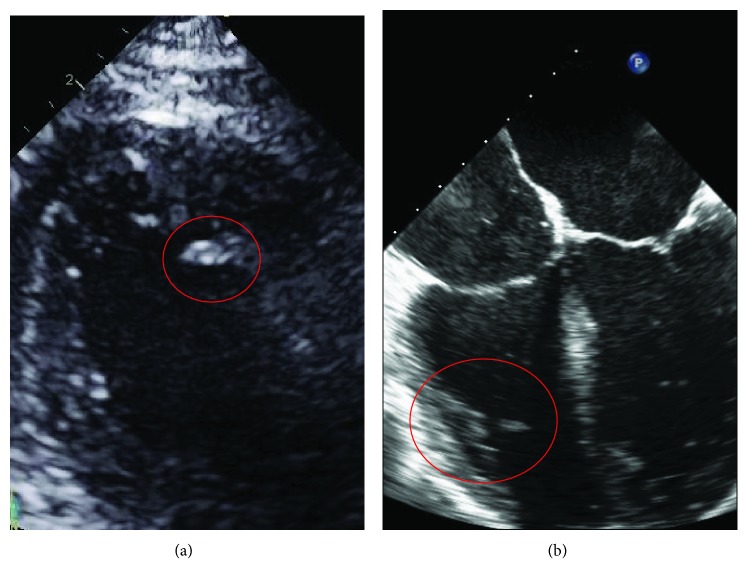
Zoomed-in transthoracic apical 4-chamber view of left ventricular (a) and transesophageal 4-chamber view of right ventricular (b) thrombi.
